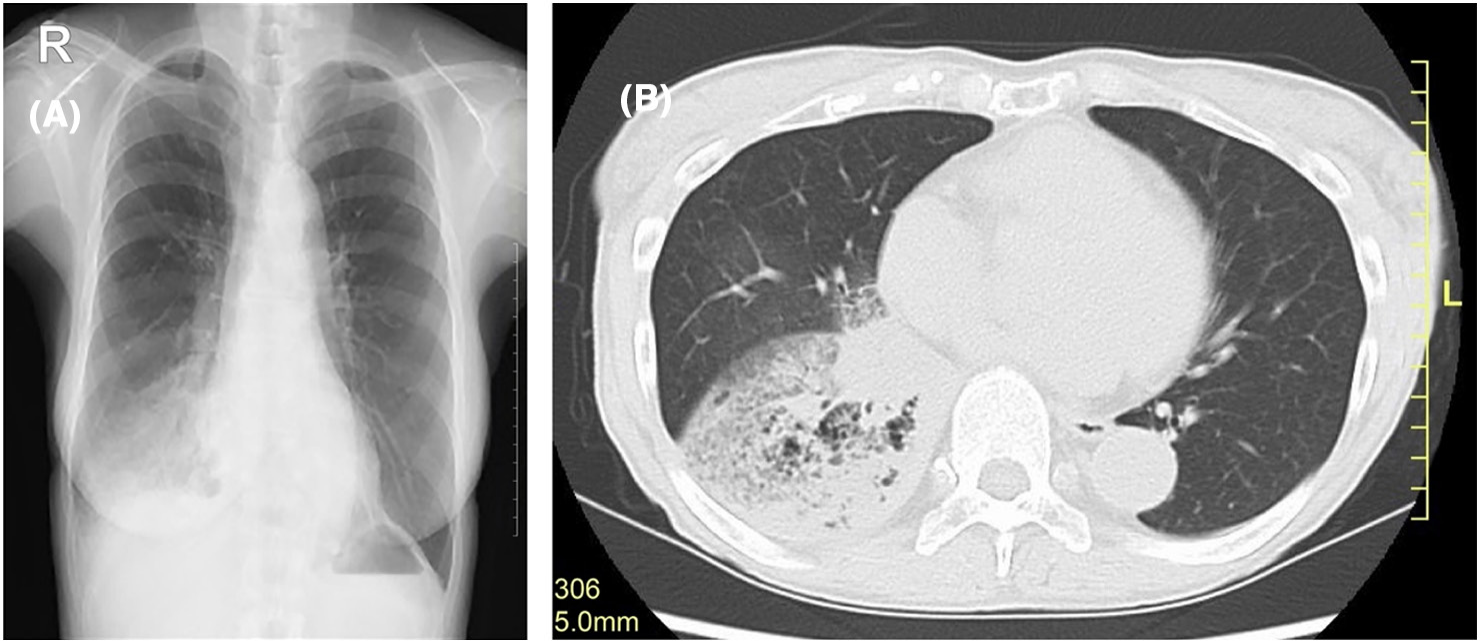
Chest radiograph (frontal view) and chest computed tomography findings. (A) An extensive infiltrative shadow is visible in the right lower lung field. (B) The entire right lower lobe had frosted to infiltrative changes extending 20 cm. Some bronchiectatic dilatations are visible in the inner part of the lobe, suggesting the possibility of middle lobe infiltration beyond the major fissure.
